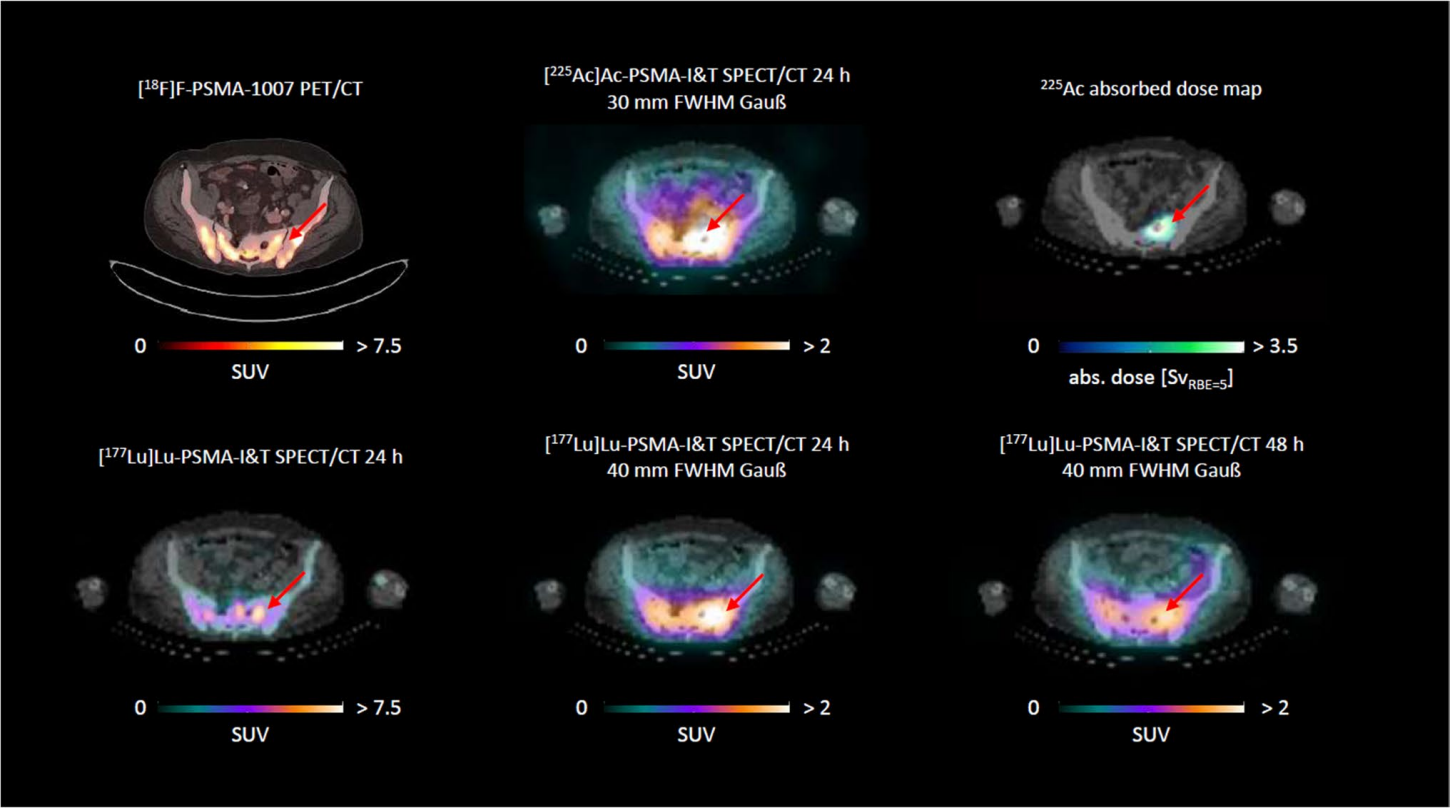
Transversal slices of the pelvic region for patient 5; the pre-therapeutic PSMA PET/CT is shown together with the quantitative [^177^Lu]Lu-PSMA-I&T SPECT/CT at 24 h and 48 h p. i. and the single quantitative [^225^Ac]Ac-PSMA-I&T SPECT/CT at 24 h, as well as the resulting ^225^Ac RBE-weighted absorbed dose distribution
